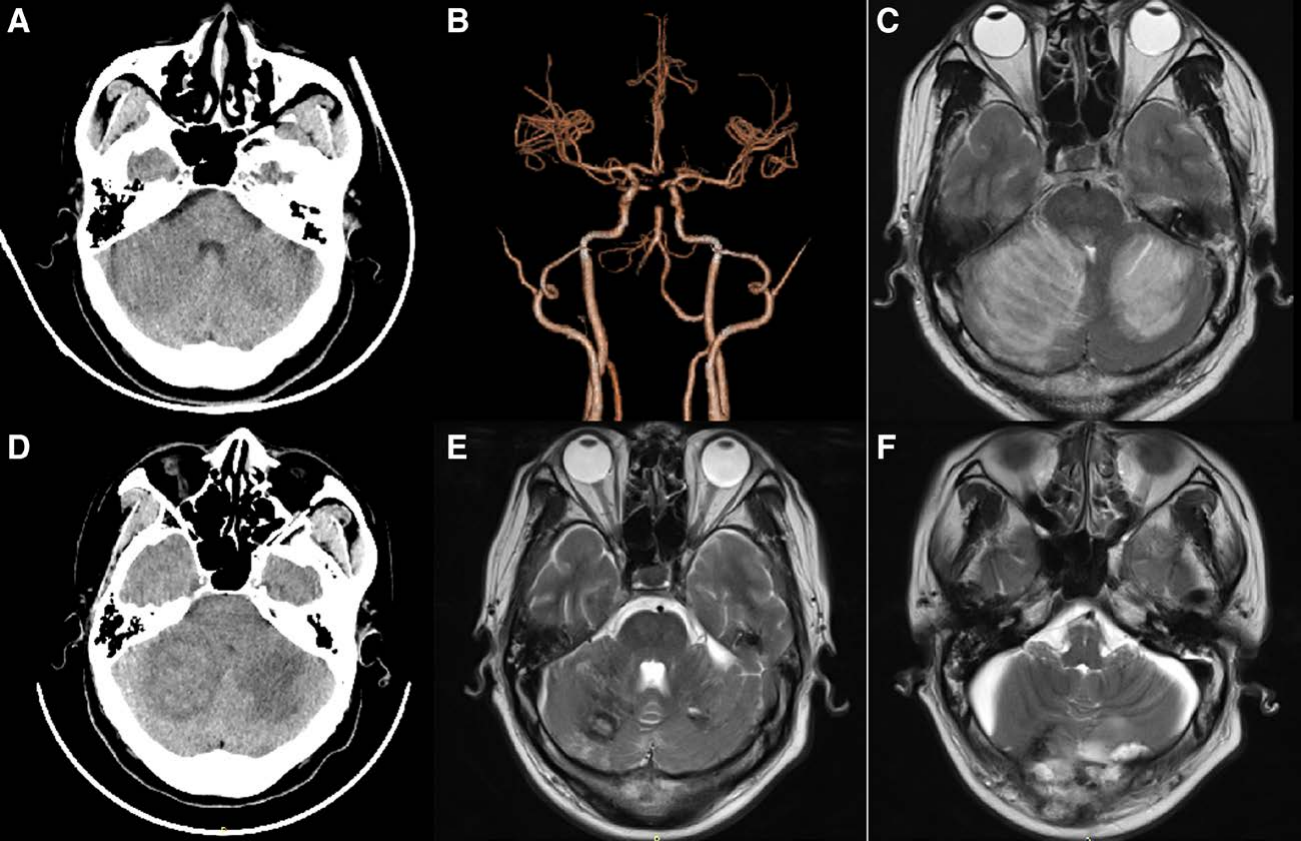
A 46-year-old man with MCI including in our study, presented with sudden dizziness and vomiting that lasted for 12 h and disturbance of consciousness that lasted for 2 h. (A) Cranial CT suggested bilateral massive cerebellar infarction at admission. (b) Cranial CTA suggested basilar artery occlusion. (C) After EVT, the patient was conscious, and cranial MRI still indicated bilateral massive cerebellar infarction. (D) On the 2nd night after EVT, loss of consciousness deepened, and cranial CT indicated cerebellar infarction with a space-occupying effect. The family consented to decompressive craniectomy. (E) At 3 weeks after craniectomy, the patient was conscious and could get out of bed and had a mRS score of 2 points. The cranial MRI indicated a bilateral cerebellar infarction that had been reduced. (F) The patient had a mRS score of 1 at discharge, and the cranial MRI indicated a further reduction in the area of bilateral cerebellar infarction. CT = computed tomography, CTA = computed tomography angiography, EVT = endovascular treatment, MCI = massive cerebellar infarction, MRI = magnetic resonance imaging, mRS = modified Rankin Scale.
